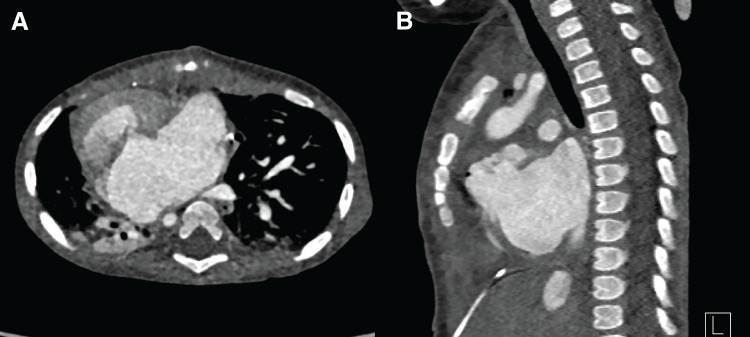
Postoperative evaluation. (A) Axial CT image demonstrating cardiomegaly without evidence of external sternal compression. (B) Sagittal CT view demonstrating adequate sternal elevation and improved mediastinal volume.
